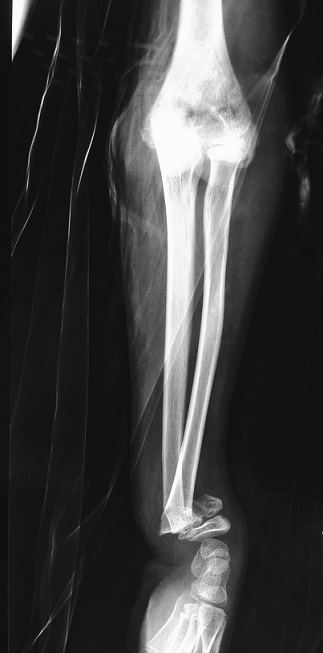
The wrist lateral radiograph showing epiphyseal separation fractures of the radius
